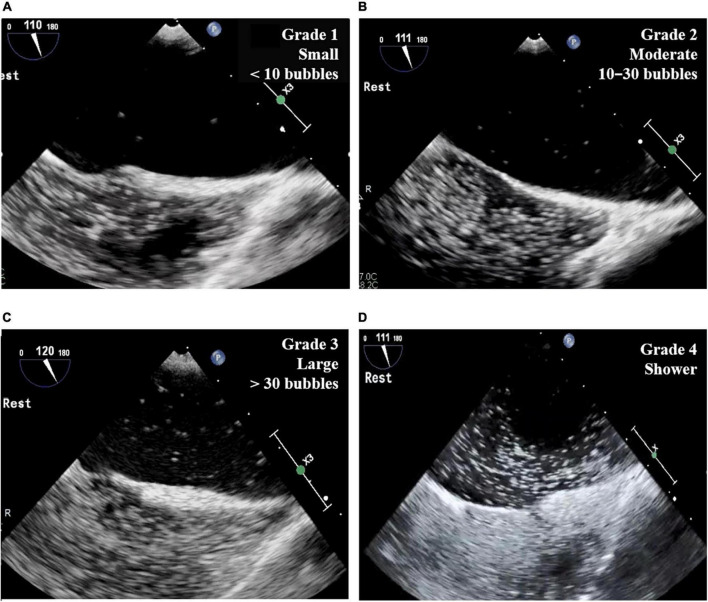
Grade of TECE with agitated saline test. (A) Grade 1 (small): fewer than 10 bubbles in LA; (B) grade 2 (moderate): 10–30 bubbles in LA; (C) grade 3 (large): more than 30 bubbles in LA; (D) grade 4 (shower): extensive contrast in LA. TECE, transesophageal contrast echocardiography; LA, left atrium.
